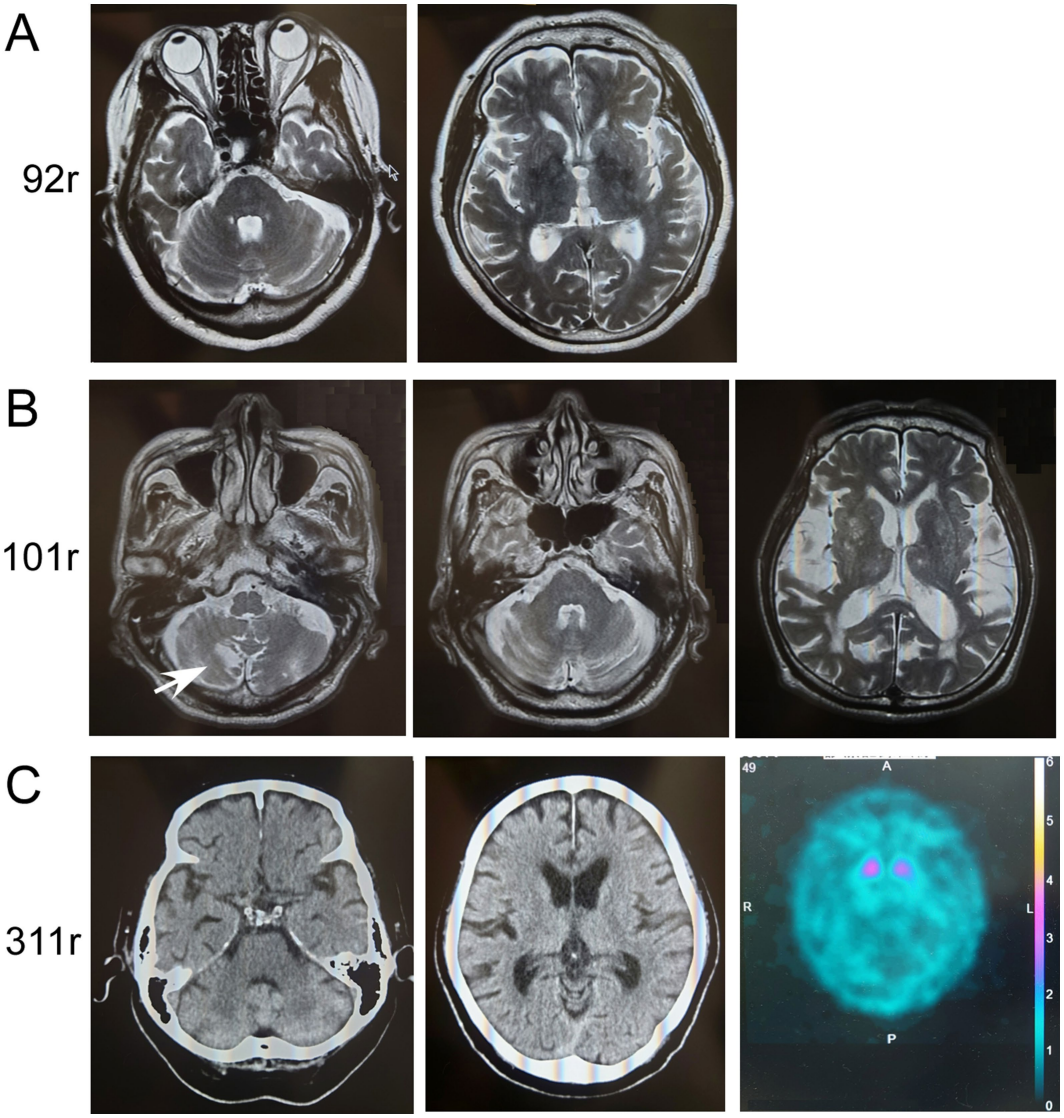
Imaging results of patients with *ATXN8OS* repeat expansions. (A) MRI of Patient 1 with 92 repeats (92r) showed no apparent atrophy of the cerebellum (upper panel) or cerebrum (lower panel). (B) MRI of Patient 2 with 101 repeats (101r) showed asymptomatic infarction of the cerebellum (arrow) and slight non-specific atrophy of the cerebellum or mild atrophy of the cerebrum with chronic ischemic lesions. (C) Computed tomography (CT) of the head in Patient 3 with 311 repeats (311r) showed no apparent atrophy of the cerebellum (left panel) or cerebrum (right panel). Dopamine-transporter single photon emission computed tomography showed marked reduction of the uptakes in the striatum. The specific binding ratio was 1.26 on the right striatum and 1.62 on the left.
